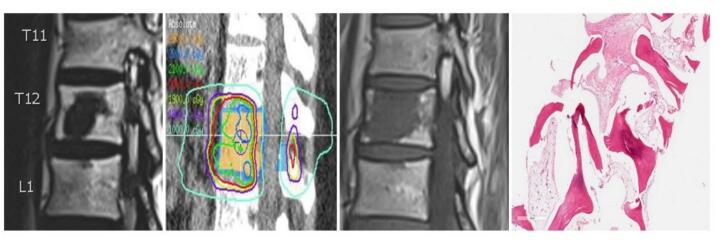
Patient treated with 24 Gy in 2 fractions with ER/PR+ oligo-metastatic breast cancer. 10 years later while on no endocrine therapy a follow-up MRI showed this sudden T1 signal change within the treated vertebral body. The biopsy showed fibroses and no active tumor.
